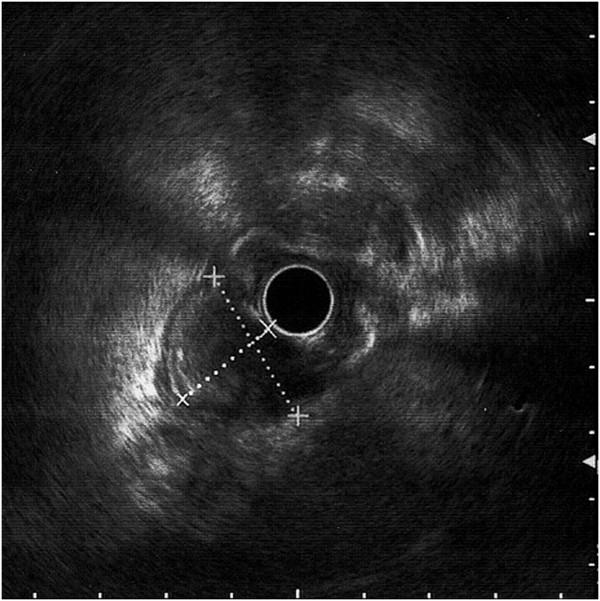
Endoscopic ultrasound (EUS) of the inflammatory myofibroblastic tumor (IMT). An oval hypoechoic mass, 25 × 17 mm in diameters was identified arising from the muscularis propria layer.
